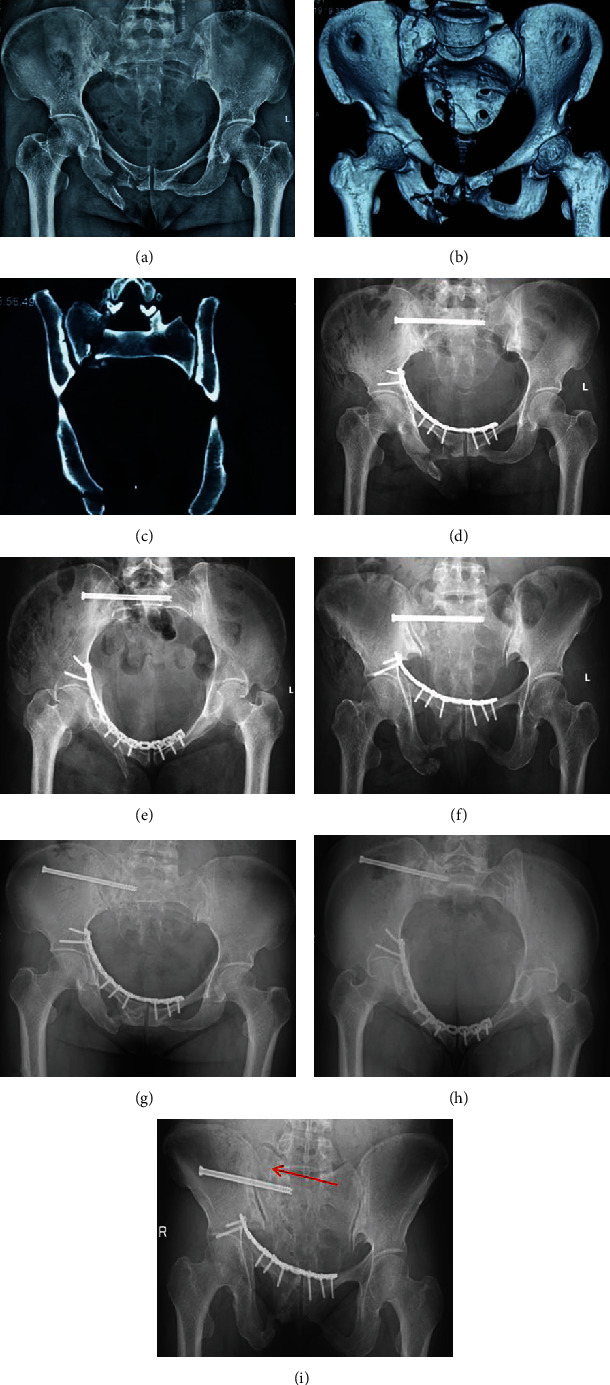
Patient, female, 41, with pelvic fractures, front ring plate fixation. The posterior ring was fixed with a single sacroiliac screw. (a) Anteroposterior view of pelvic fracture. (b) 3D CT preoperative pelvic factures for Tile C1.3 type, unilateral vertically unstable pelvic ring. (c) Preoperative CT scan in pelvic ring through the sacrum fracture, vertically displaced. (d) Anteroposterior radiograph of the pelvis immediately after the operation showed satisfactory reduction of the fracture. (e) Inlet radiograph of the pelvis immediately after surgery. (f) Outlet radiograph of the pelvis immediately after surgery. (g) Anteroposterior radiographs of the pelvis 18 months after surgery showed fracture healing. (h) A radiograph of the pelvic inlet 18 months after surgery showed fracture healing. (i) A radiograph at the exit of the pelvis 18 months postoperatively showed healing of the fracture and loss of reduction at the arrow.
